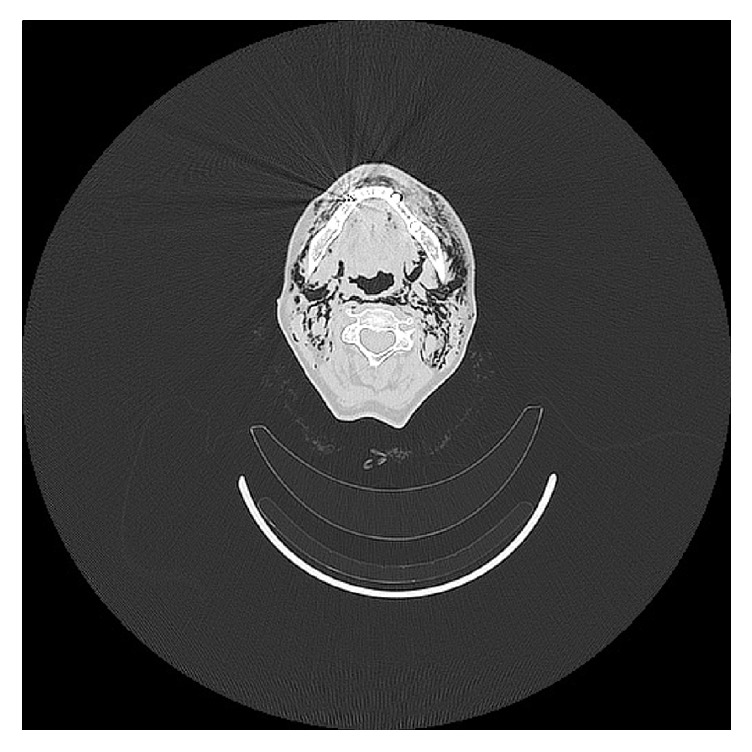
Computed tomographic axial view of the emphysema in the mandibular region.
